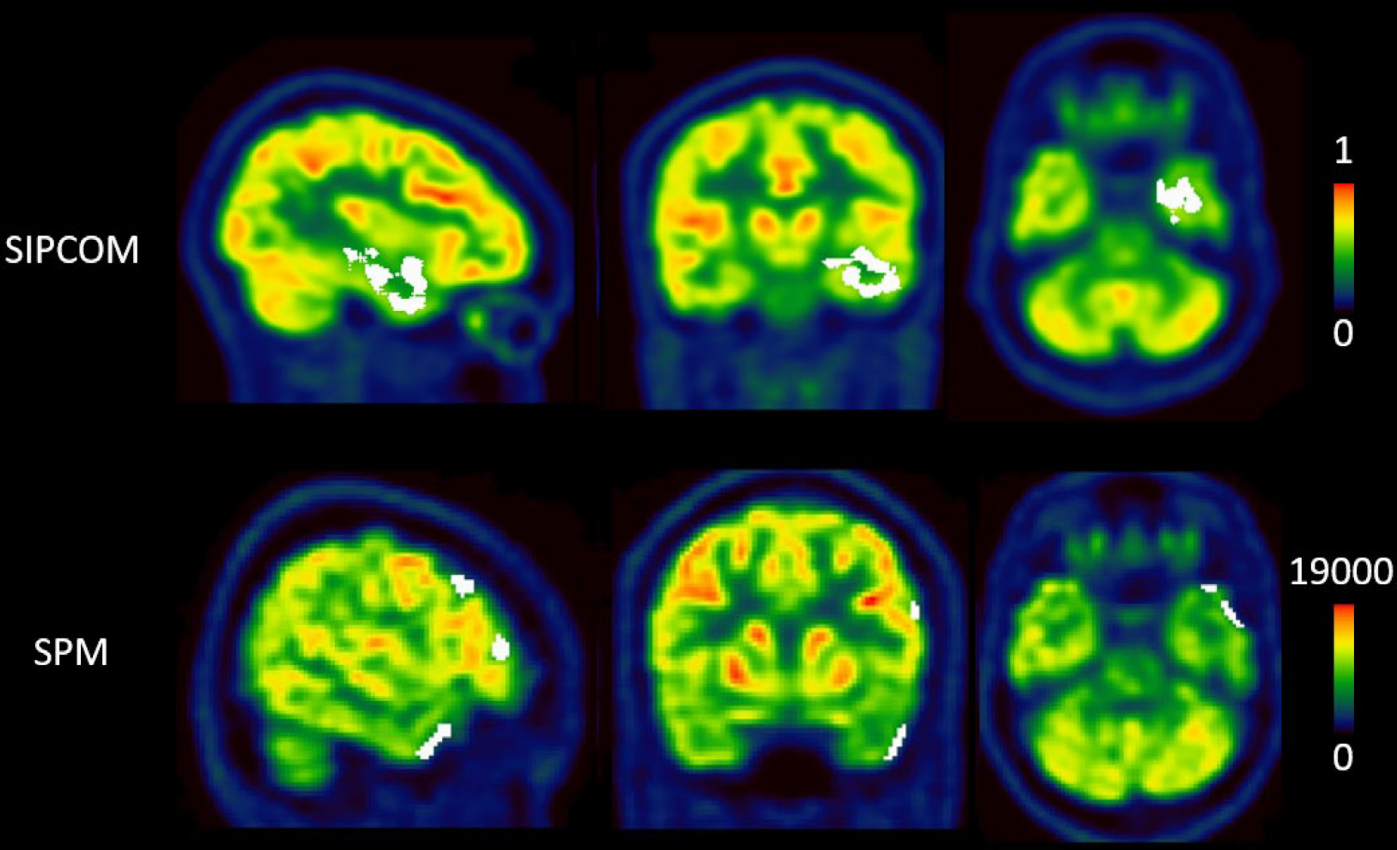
Result of a concordant case between statistical parametric mapping (SPM) and Subtraction Interictal PET Co‐registered to MRI (SIPCOM) analysis (patient #5). The first row displays the [^18^F]FDG PET superimposed with an abnormal cluster (in white) defined using SIPCOM. The second row displays [^18^F]FDG PET superimposed with abnormal clusters defined using SPM. Cluster size and localisation did not overlap between analyses. The frontal clusters seen with SPM (false positives) likely reflect the patient's individual anatomy and are therefore not seen with SIPCOM.
